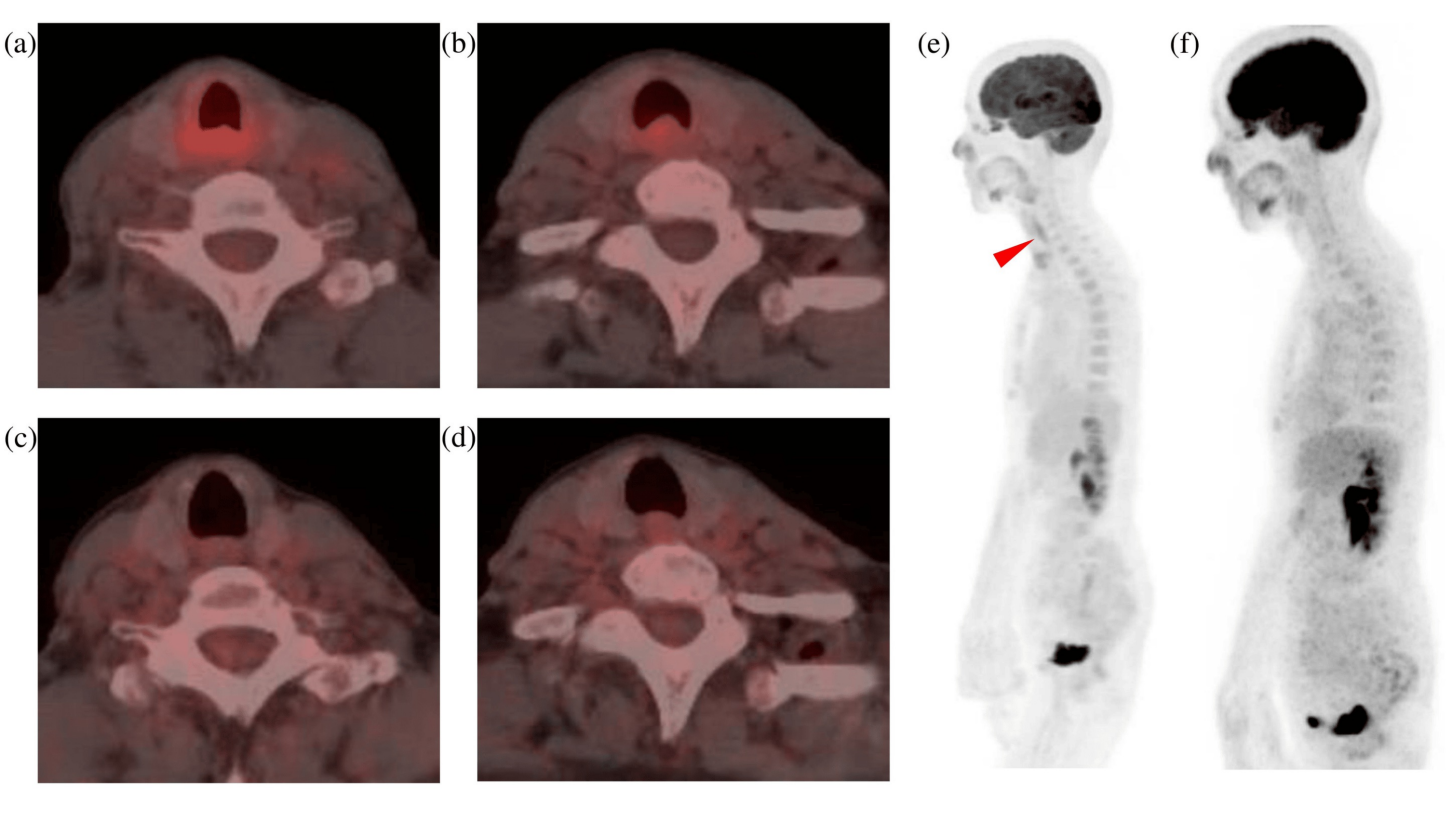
18F-FDG PET/CT images before and after four courses of chemotherapy (a,b,e) Before chemotherapy. The red arrow indicates increased FDG uptake in the posterior tracheal wall; (c,d,f) After chemotherapy FDG: fluorodeoxyglucose
